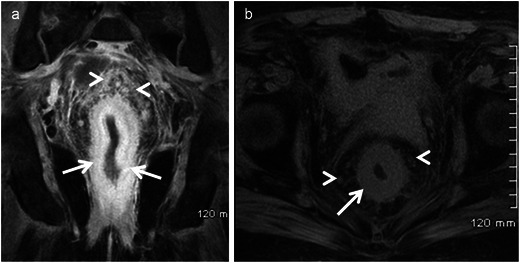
Diffuse cavernous haemangioma of the rectum in a 21-year-old man presenting with painless rectal bleeding. a Coronal and b axial fat-suppressed contrast-enhanced T1-weighted MRI reveal enhancing concentric rectal wall thickening (*arrows*) with enhancing serpiginious structures (*arrowhead*) in the mesorectum
